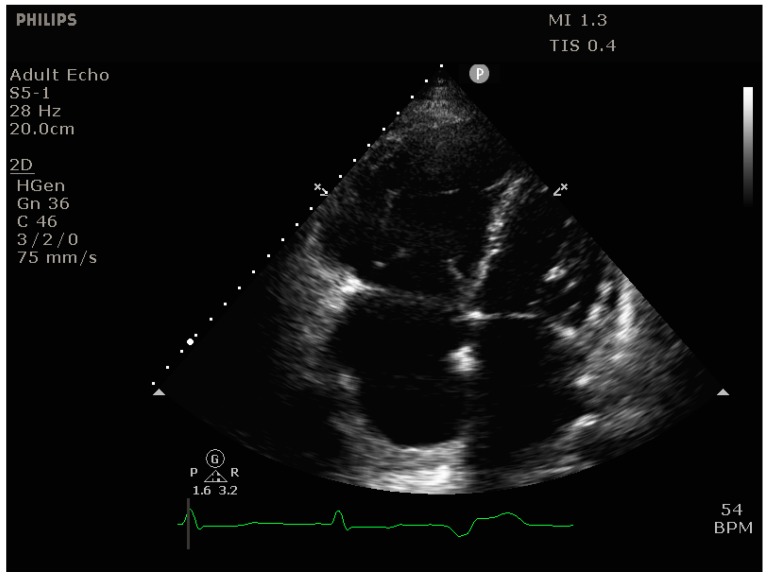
Transthoracic echocardiogram (apical 4-chamber view) showing massive right ventricular dilation (RV diameter > LV diameter).
